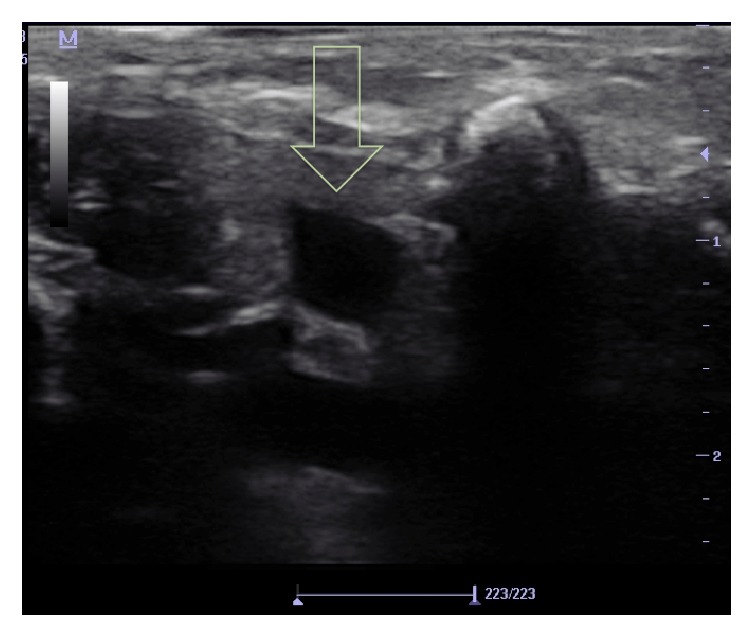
The ultrasound image of the subclavian vein. Yellow arrow is showing the subclavian vein.
